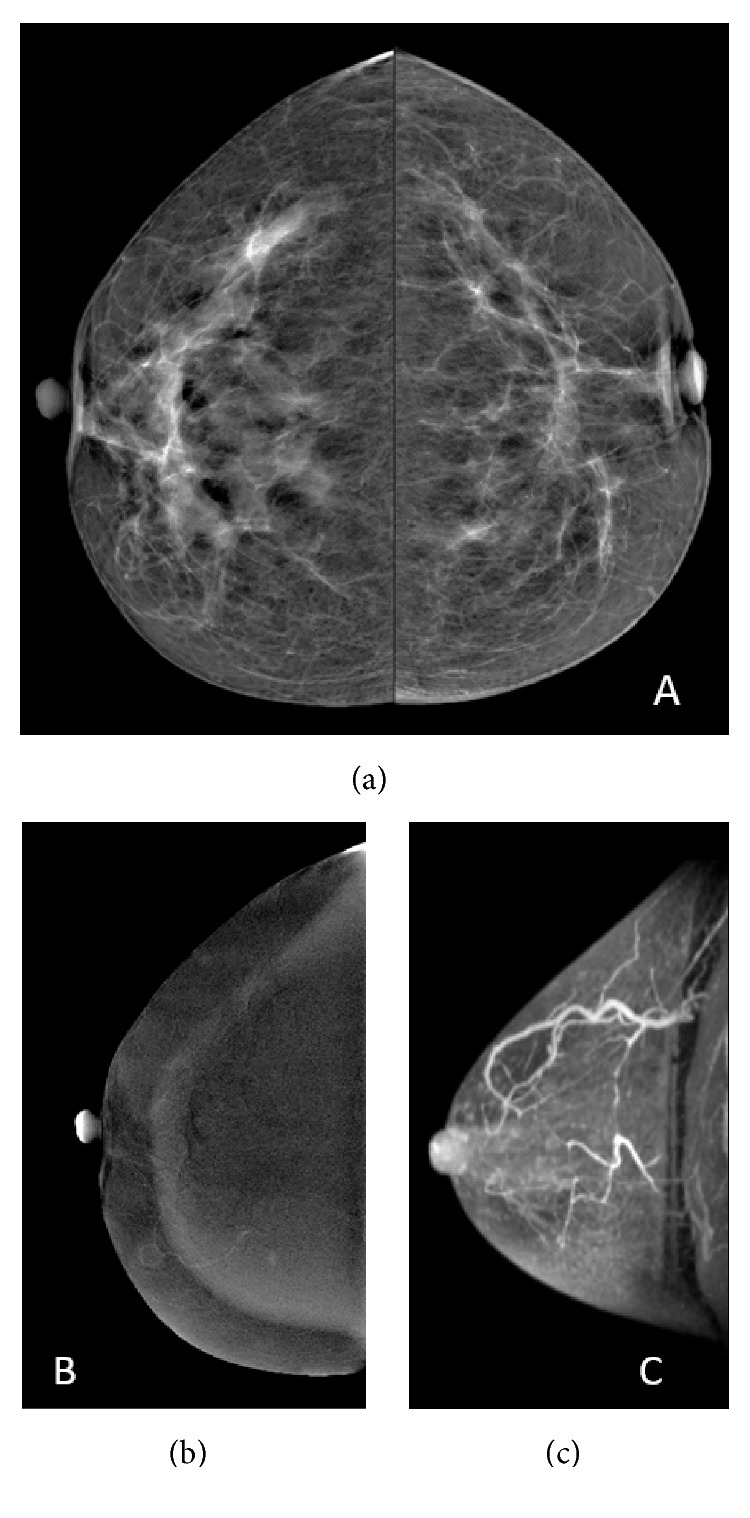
52-year-old woman. Invasive ductal carcinoma with pathologic complete response. Focal asymmetry is detected in the upper outer quadrant of the right breast (a). Contrast-enhanced mammography (b) and magnetic resonance imaging (c) without detectable lesions.
